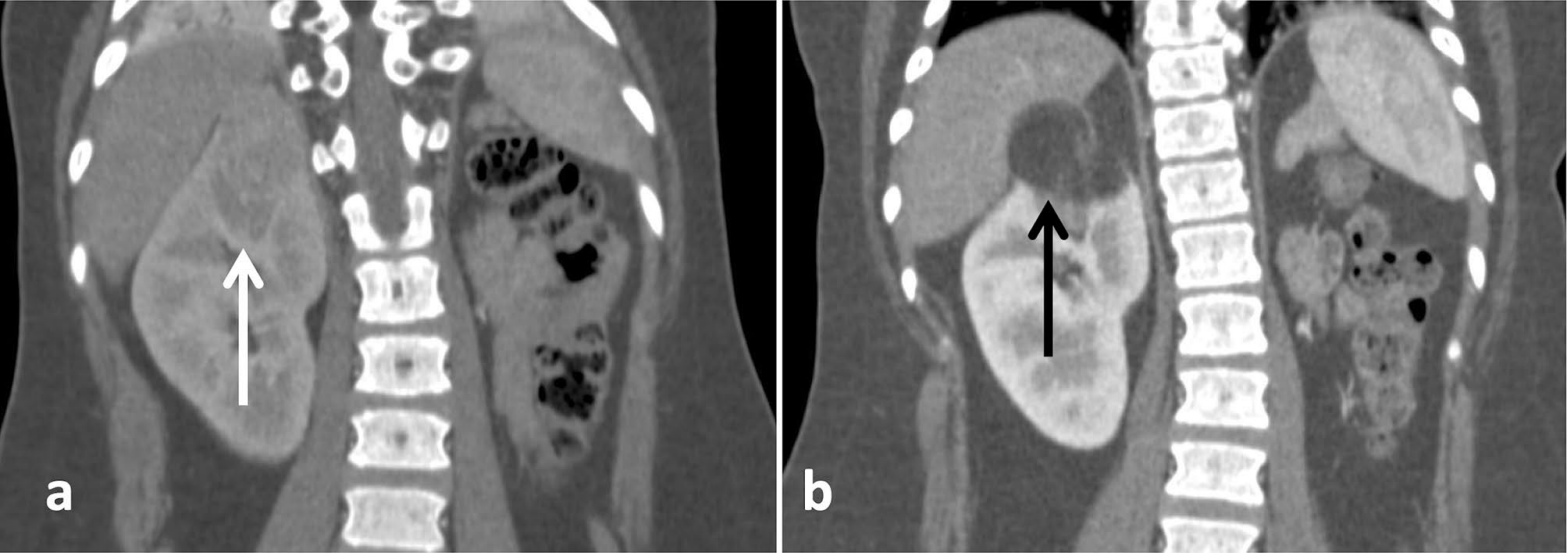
Liposubstitution in angiomyolipoma in response to treatment with sirolimus. Coronal corticomedullary-phase CT images obtained (a) before treatment and (b) after treatment show the effect of sirolimus treatment on a fat-poor AML in the upper pole of the right kidney (white arrow), revealing the appearance of a fat-rich tumor (black arrow) in substitution to the previous fat-poor pattern.
